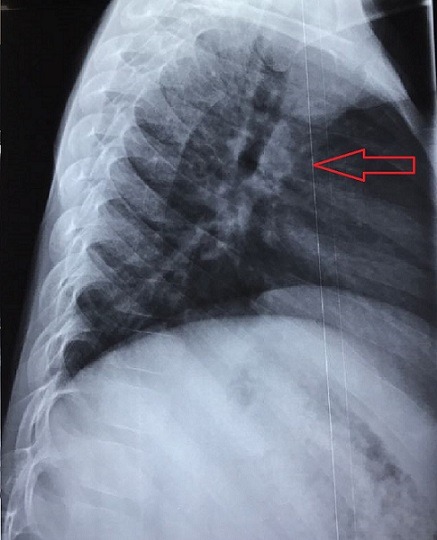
Radiographie standard de l'épaule gauche de profil à l'admission montrant des lésions lytiques au niveau de l'omoplate gauche
